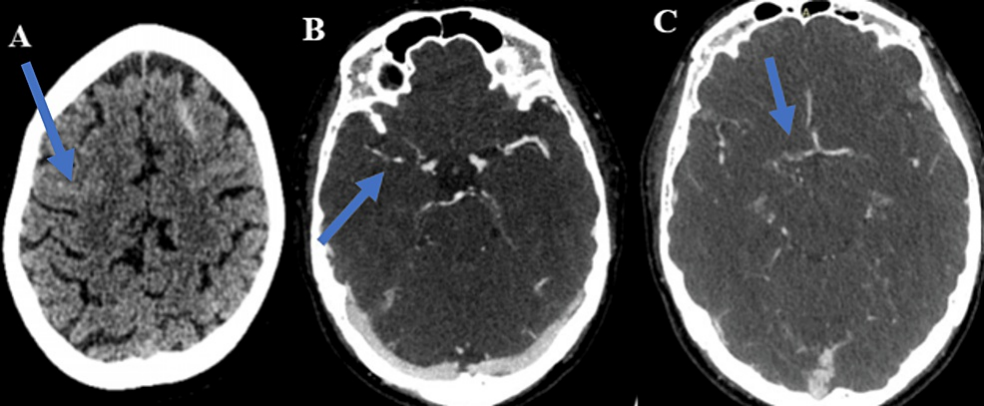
A 52-year-old woman with sudden onset of severe headache. (A) Unenhanced CT shows focal SAH in both frontal regions (right side - blue arrow). (B-C) CT angiogram shows narrowing (blue arrows) in the right middle cerebral artery (B) and right A1 segment of the anterior cerebral artery (C). Follow-up CT angiogram after five months (not shown) shows resolution of the narrowing. CT: computed tomography; SAH: subarachnoid hemorrhage
